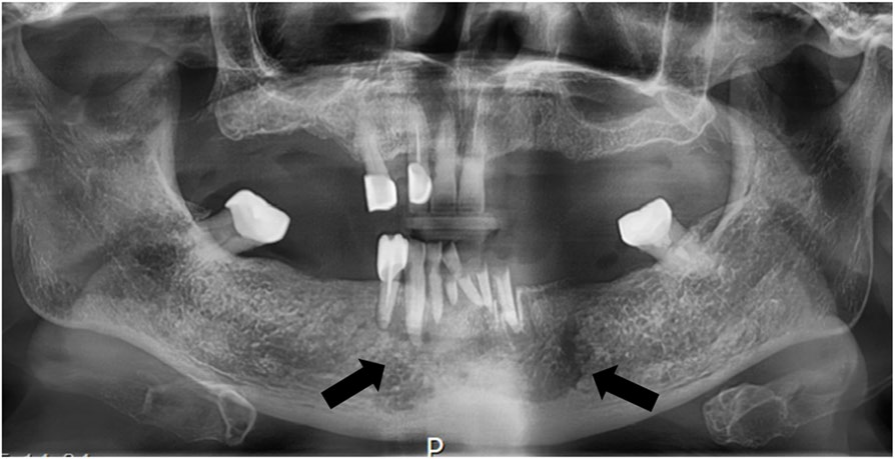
Panoramic radiograph on the first visit. Radiolucent periapical lesion around the #41-33 region and ill-defined bone loss
